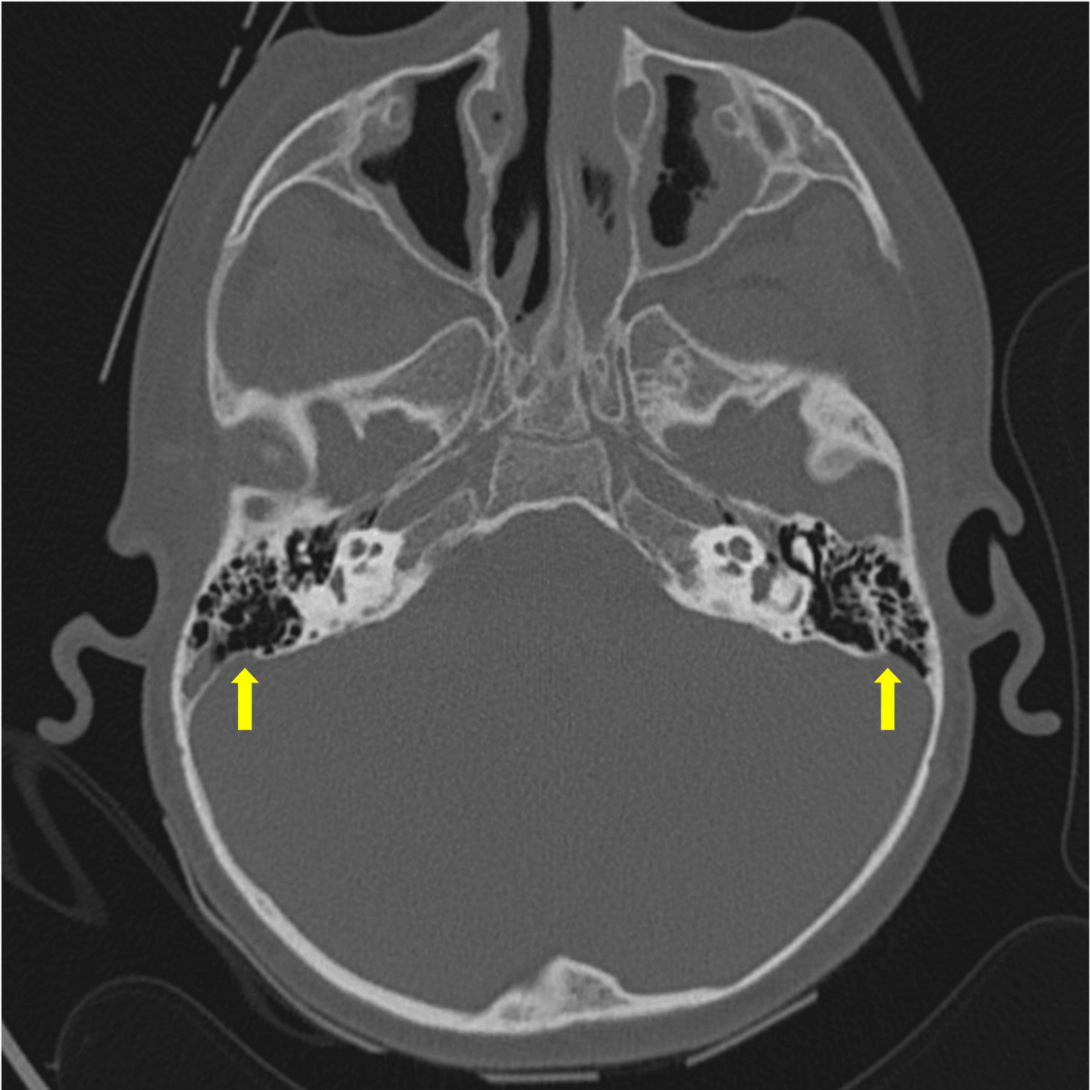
Temporal bone computed tomography: Both mastoid cavities are well-pneumatized, and the middle ear shows no signs of occupation.
